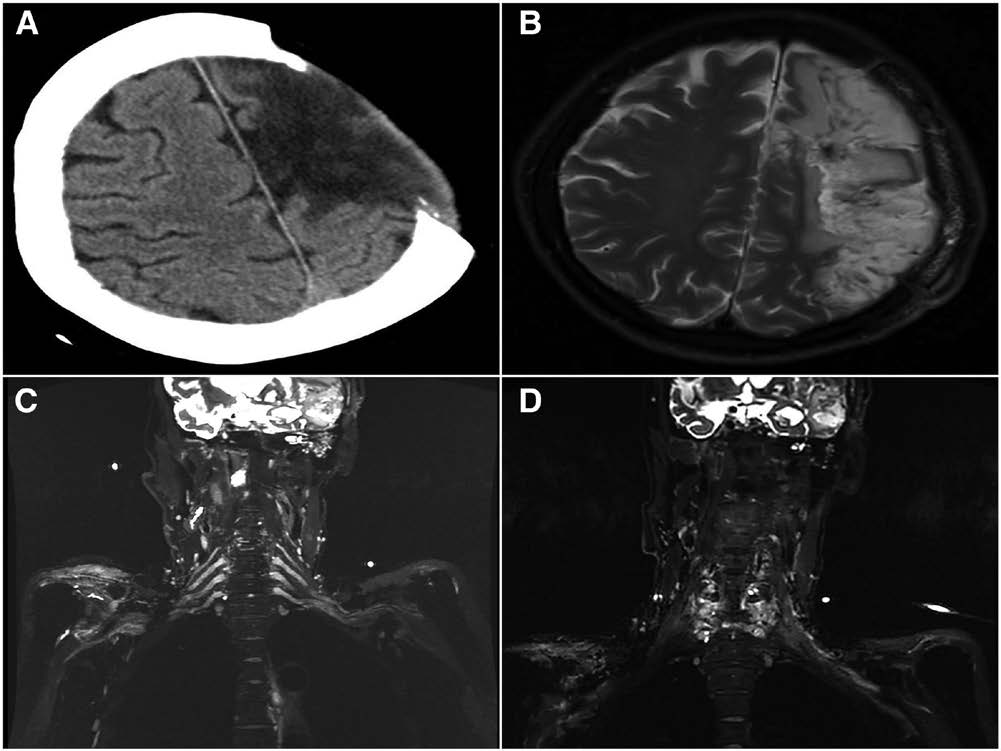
Are preoperative images of a patient who underwent PSCC7 approach. (A) Is preoperative cranial CT scan showing extensive infarction in the left hemisphere. The patient underwent decompressive craniectomy because of the large infarction. (B) Is a preoperative MRI showing significant functional area damage, notably in the motor area for the upper limb on the left side. (C and D) Preoperative MRI of the cervical spine showing the nerve roots of C4 to C7 and the surround tissues.
